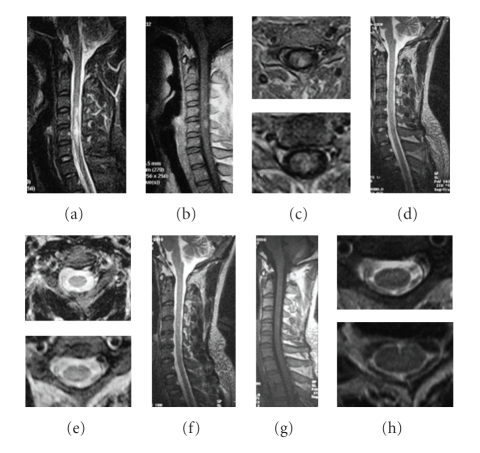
A 30-year-old male suffered from left paraparesis and paresthesias for 1 month. MRI of the cervical spinal cord revealed a demyelinating lesion in T2-weighted images extending from C4 to C7. Pulse methylprednisolone and IVIG were initiated with no resolution. The MRI changes are as follows: (a) sagittal T2-weighted image reveals a high intensity signal from C4 to C7 before ATT regimen, (b) sagittal T1-weighted MRI showing light thickening of the cord with subtle intraparenchymal hyperintensity, (c) the left local lesions on axial FLAIR sequences, (d) the hyperintensity resolved following 6 months of ATT, (e) no lesion is visible on axial T2-weighted images, and (f)–(h) no lesion is visible on follow-up MRI after 2 years of treatment.
